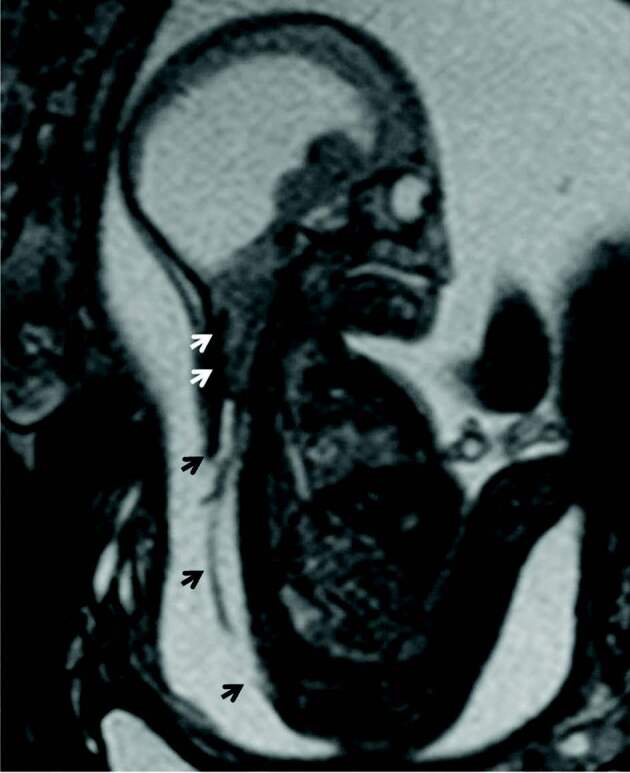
Sagittal T2 HASTE from fetal MR imaging demonstrates severe cerebellar ectopia (white arrows) and large defect in the posterior arch of the thoracic and lumbosacral vertebrae (black arrows). Findings are consistent with Chiari II malformation.
